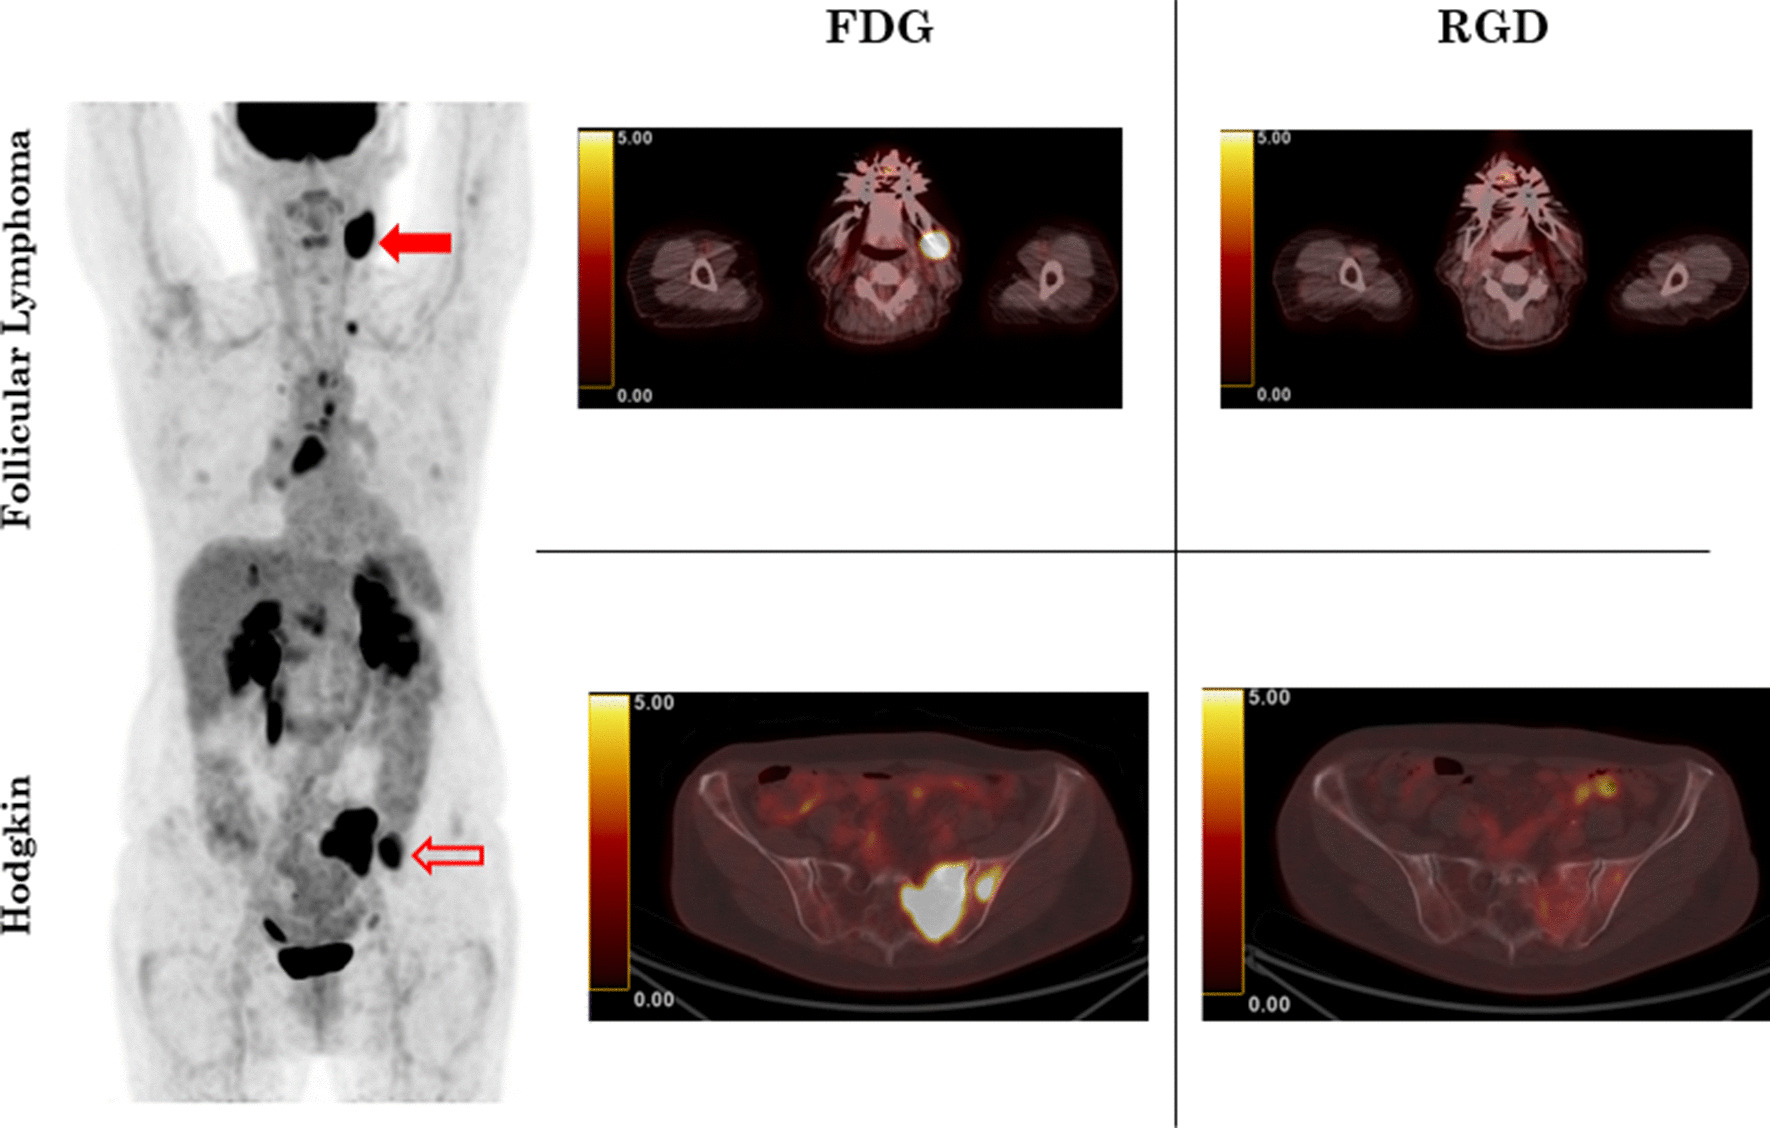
FDG maximum intensity projection (left) in patient presenting with synchronous biopsy-proven follicular (full red cross) and Hodgkin (empty red cross) lymphoma. On axial PET/CT (middle and right column), both lesions had significant FDG uptake (middle column). No RGD uptake in follicular lymphoma (right column, upper row). Significant RGD uptake in Hodgkin lymphoma (right column, bottom row)
